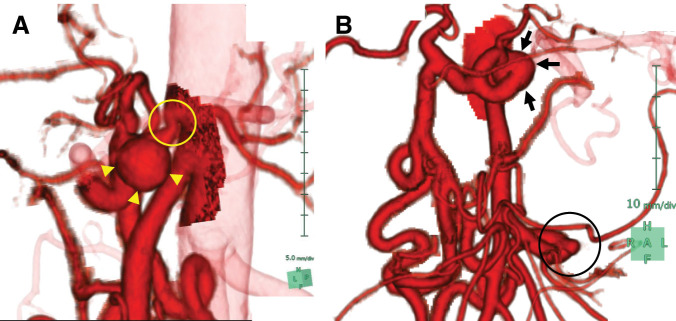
3D CT reconstruction findings at initial presentation. (A) Lateral view, confirming the hook-shaped stenosis (circle) and aneurysm of the CA (arrowheads). (B) Anterior view, showing the aneurysms of the CA (arrows) and inferior PDA (circle), with prominently developed collateral arcades from the superior mesenteric to the gastroduodenal arteries. CA, celiac artery; PDA, pancreaticoduodenal artery
